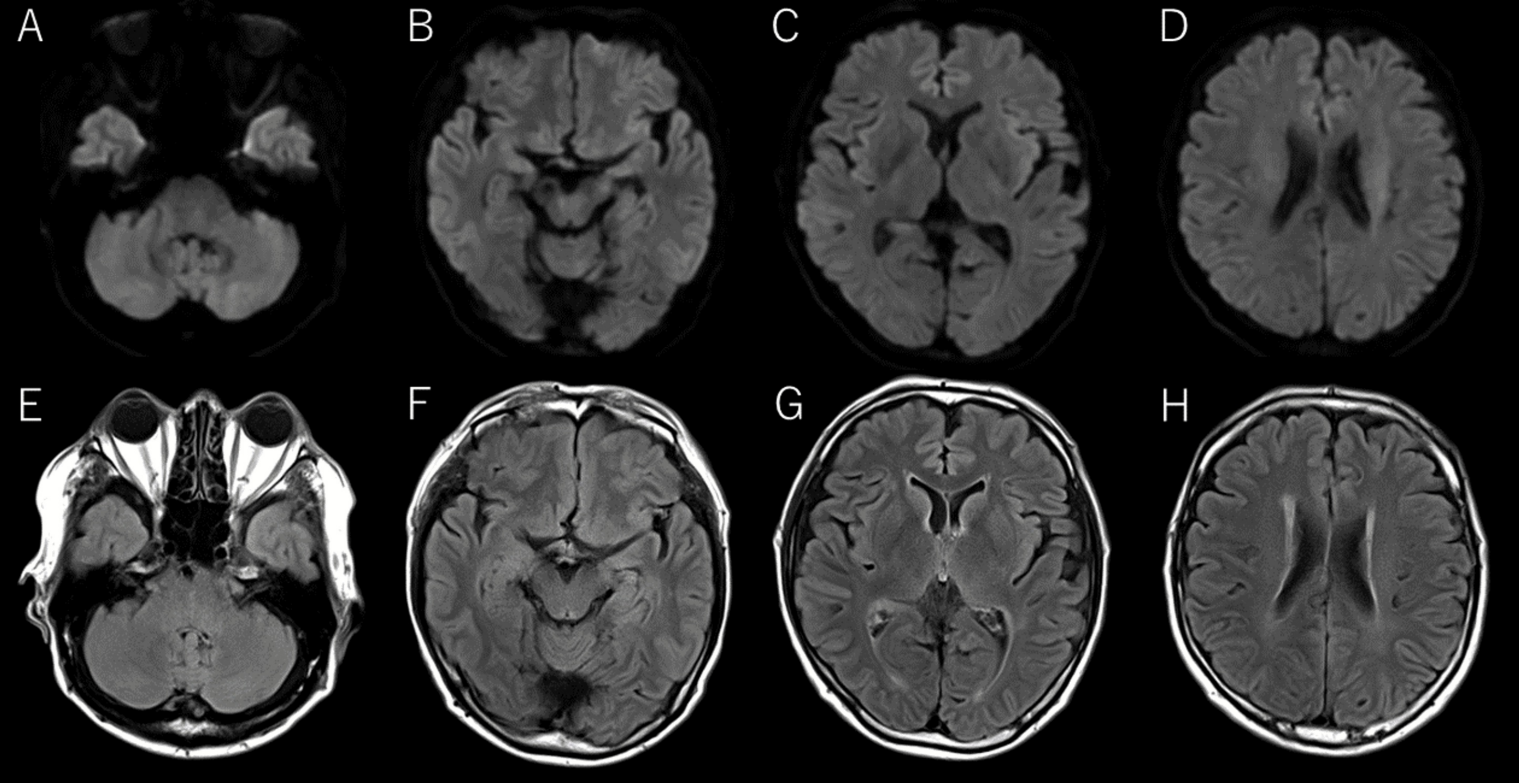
Initial brain imaging results The initial brain magnetic resonance imaging with diffusion-weighted imaging sequences (A-D) and fluid-attenuated inversion recovery sequences (E-H) showed no evidence of intracranial hemorrhage, brain tumor, or cerebral edema as a cause of the patient's headache.
